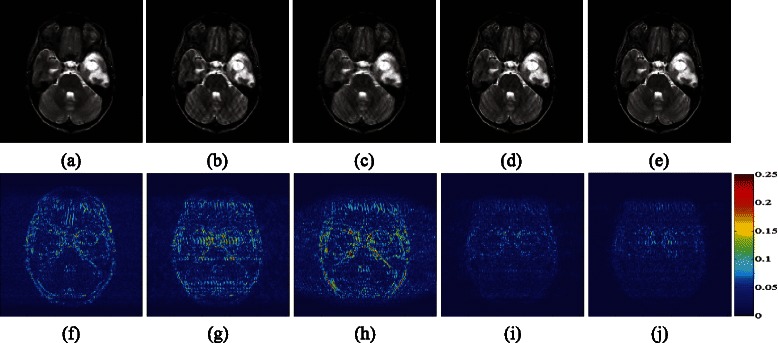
Reconstructed MBA_T2_slice006 under Cartesian undersampling scheme at 0.35 sampling rate. (a)–(e) Reconstructed images from DLMRI, MRSFLCT based CS MRI, LORAKS, PANO and LSECSMRI, (f)–(j) difference images between fully sampled MR image and images in (a)–(e) with gray scale of [0,0.25], respectively. PSNRs of them are 30.95dB, 30.25dB, 28.33dB, 35.21dB and 3
